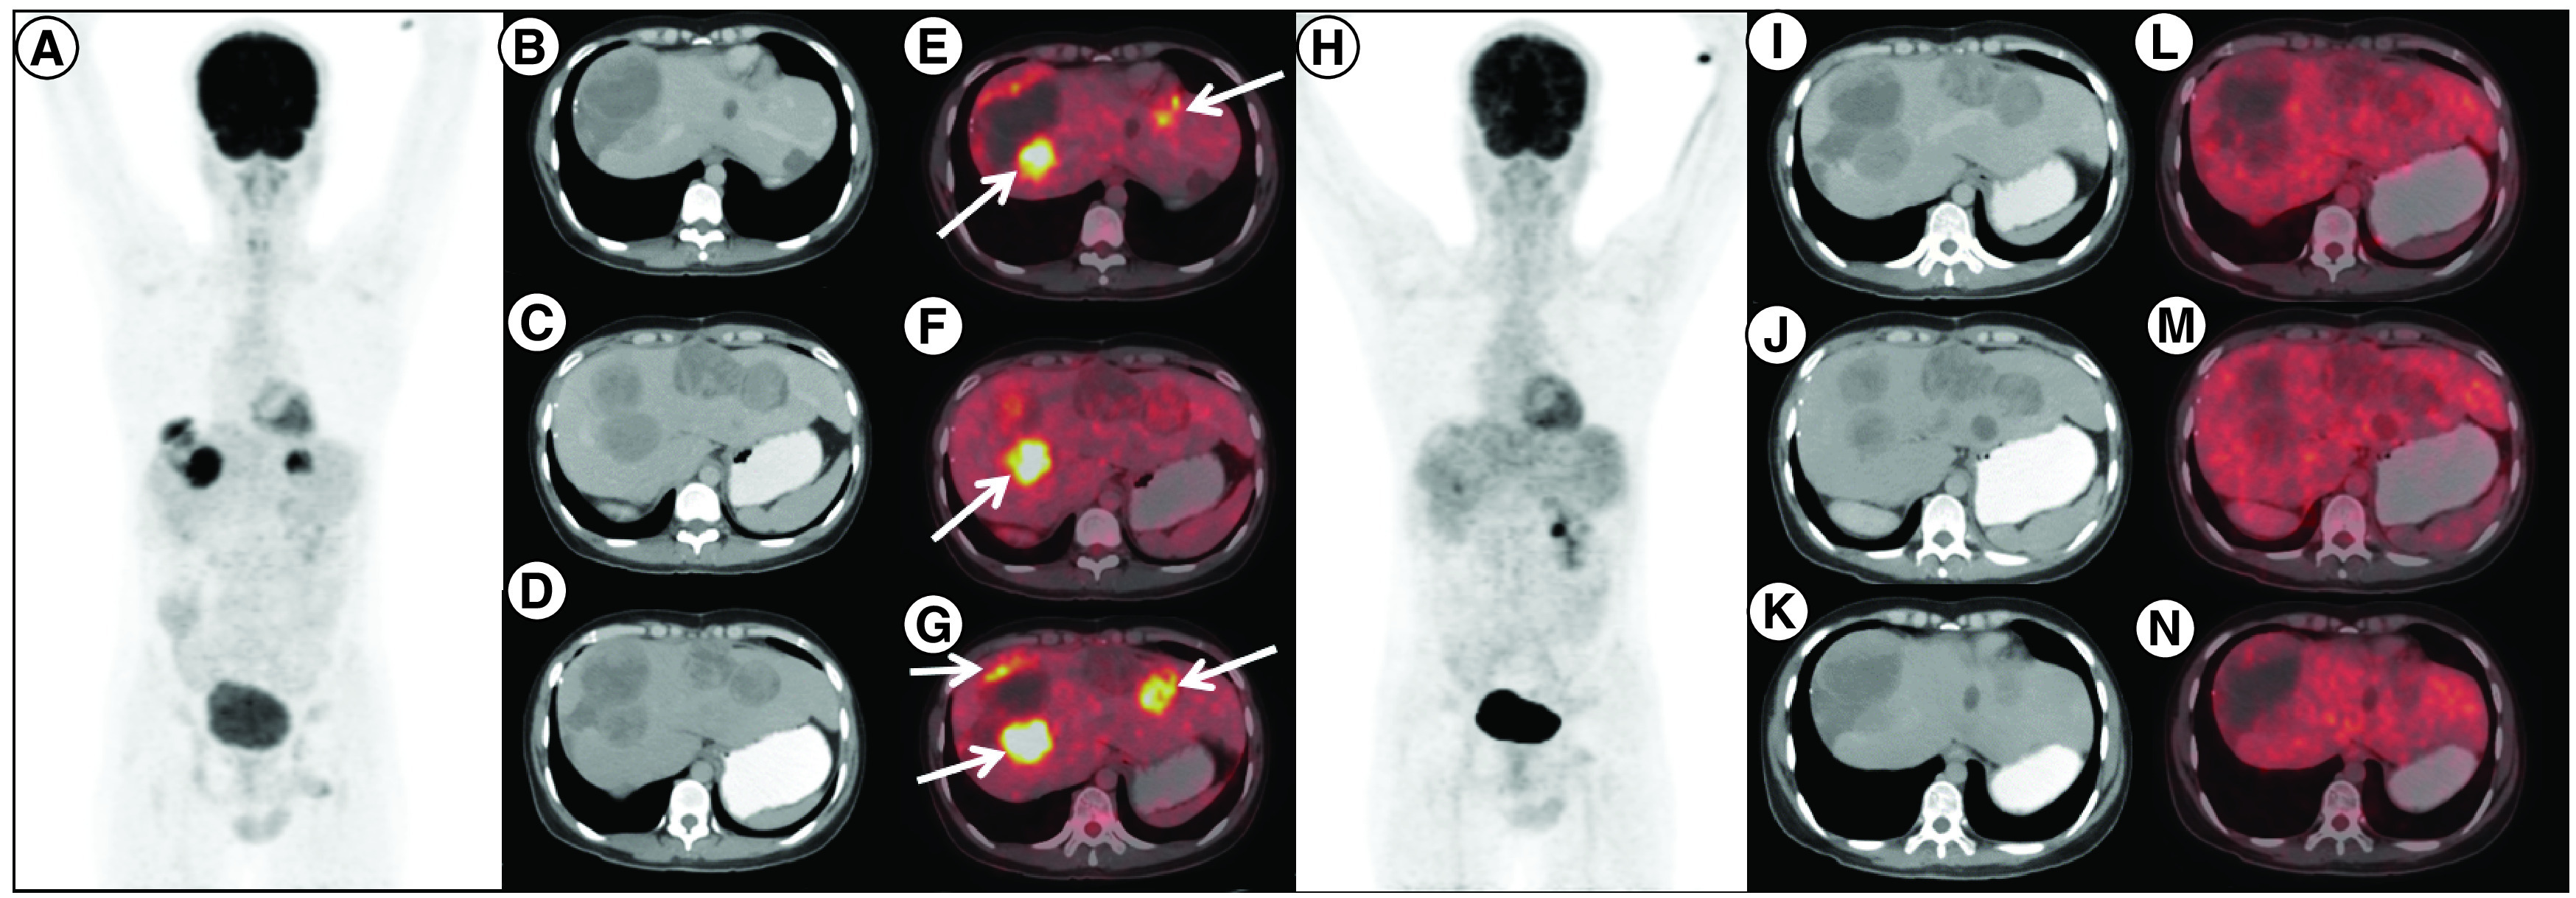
Response to imatinib, sunitinib and regorafenib therapy in case 1. ^18^F-Fluorodeoxyglucose (FDG) positron emission tomography/computed tomography (PET/CT) done post imatinib, sunitinib and regorafenib therapy in patient A. (A) maximum intensity projection, (B, C & D) axial contrast enhanced CT and (E, F & G) fused PET/CT images showing multiple solid cystic masses with increased FDG uptake in the enhancing solid component (arrows) suggestive of metabolically active liver metastases. Follow-up ^18^F-FDG PET/CT done 3 months after initiation of avapritinib therapy, which revealed resolution of metabolic activity in the corresponding lesions (H–N) with hypodense/cystic changes in the previously enhancing lesions, suggestive of complete metabolic response.
